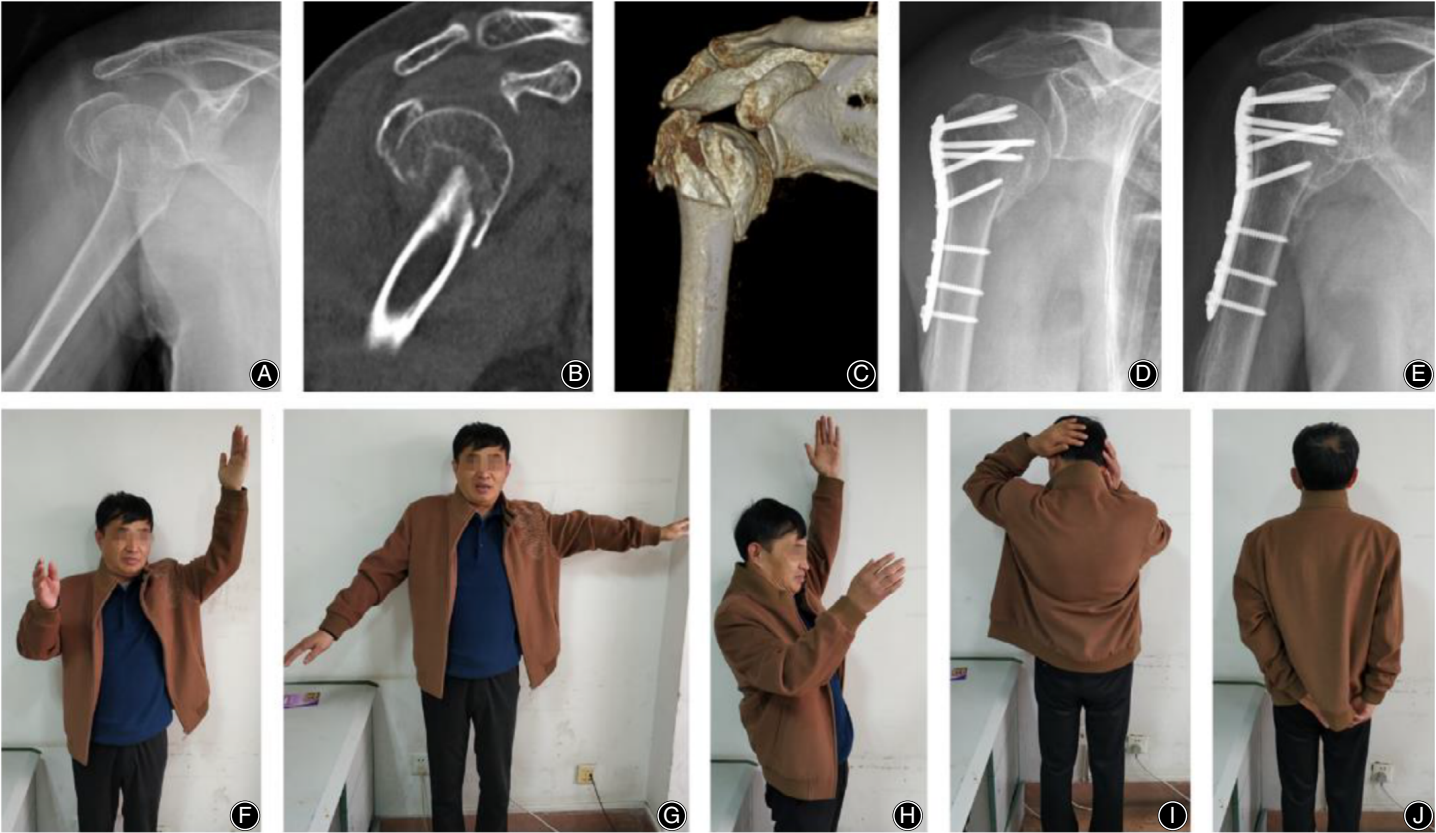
PHILOS fixation alone in a 65‐year‐old male patient with a four‐part proximal humeral fracture on the right side. (A) X‐ray film before surgery; (B) CT before surgery; (C) 3D CT before surgery; (D) X‐ray film after surgery; (E) X‐ray film 3 months after surgery; (F‐J) Function of patient's upper arm at the last follow‐up.
